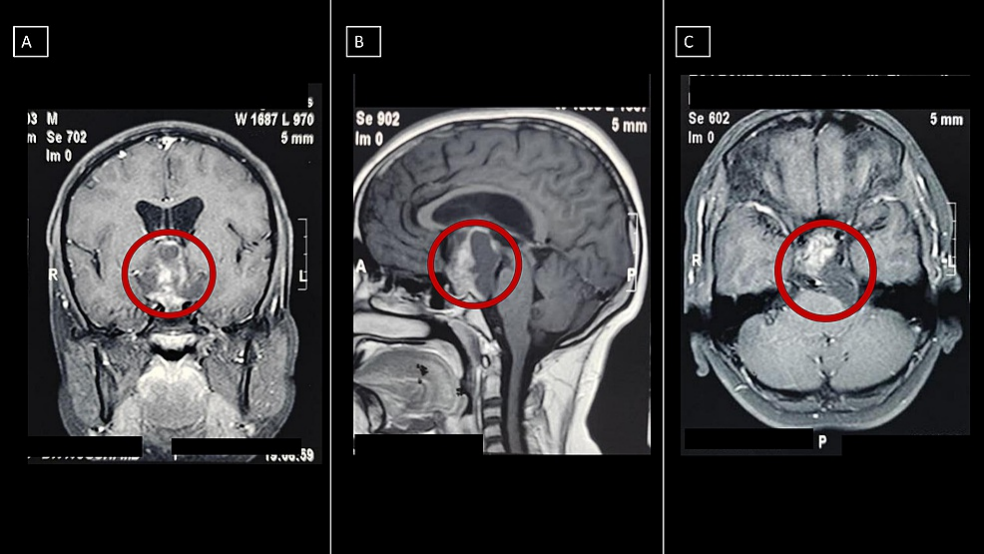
Preoperative contrast-enhanced MRI A: Preoperative contrast-enhanced coronal MRI showing a heterogeneously enhancing lesion in the sellar/suprasellar region; B: preoperative contrast-enhanced sagittal MRI showing the solid cystic nature of the sellar/suprasellar lesion with enhancing solid portion and non-enhancing cystic portion; C: preoperative contrast-enhanced axial MRI showing the solid cystic lesion centred in the sellar/suprasellar region with extension of the cystic component posteriorly into the left cerebellopontine (CP) angle cistern, causing effacement of it and causing mass effect on the left brainstem The red circle marks the location of the lesion on the MRI
